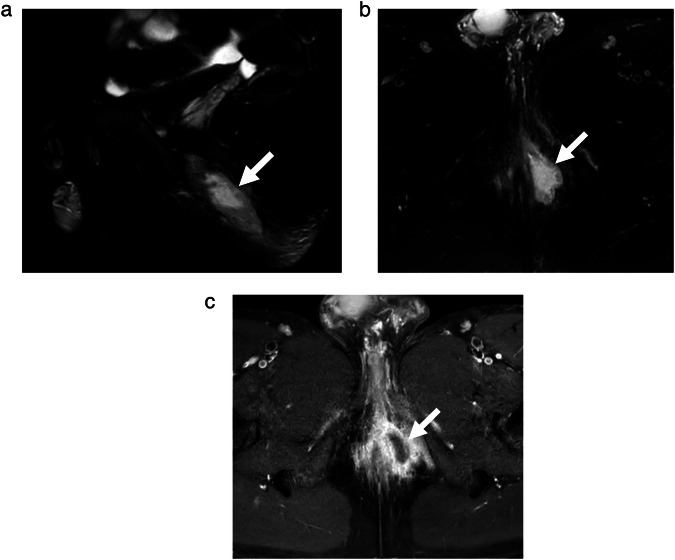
15-year-old boy with perianal Crohn’s disease. MRI pulse sequences include (a) sagittal T2-weighted (T2W) fast spin-echo (FSE) fat-saturated (FS), (b) axial T2W FSE FS, and (c) axial postcontrast T1-weighted (T1W) FSE FS. White arrows show a perianal peripherally enhancing fluid collection, consistent with an abscess. All 13 radiologists agreed on the presence of abscess/collection in this patient
